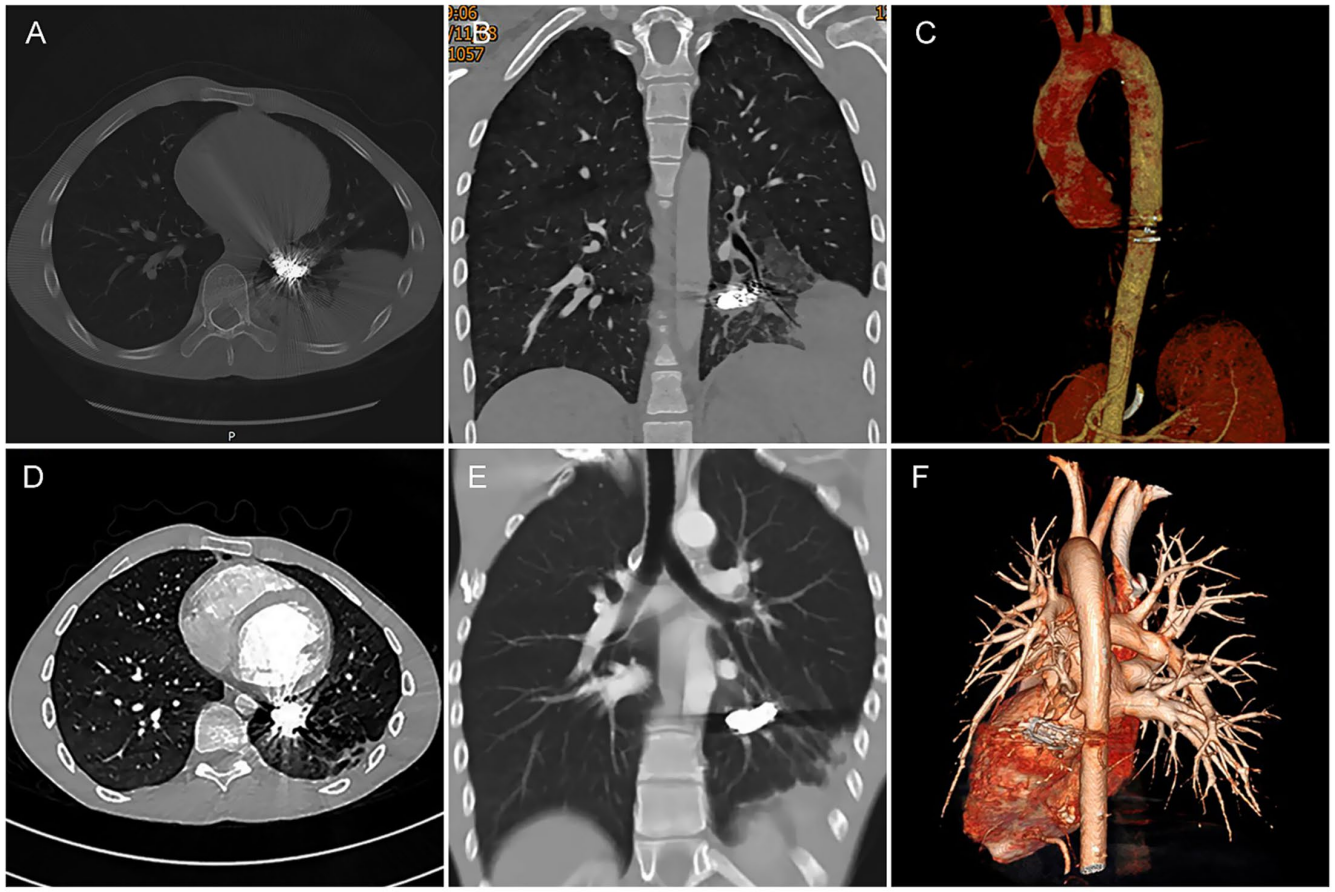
Post-embolization CT follow-up. (A−C) Three-day post-TAE CTA demonstrates: Embolic materials (coils and glue) firmly positioned within the lumen of the occluded anomalous artery. A mass-like high-density shadow was observed in the left lower lobe, surrounded by multiple patchy consolidations and ground-glass opacities. The previously abnormal artery and its branches were no longer visible, suggesting localized infarction of the left lower lobe following embolization. (D−F) One-year follow-up CTA reveals: Only minor patchy and linear opacities were noted in the left lower lobe. Mild reduction in left thoracic volume (parenchymal collapse) was observed; no significant pleural effusion, pulmonary abscess, or tissue necrosis was detected. The original aberrant systemic artery and its tributaries remained persistently invisible, indicating favorable post-embolization outcomes.
